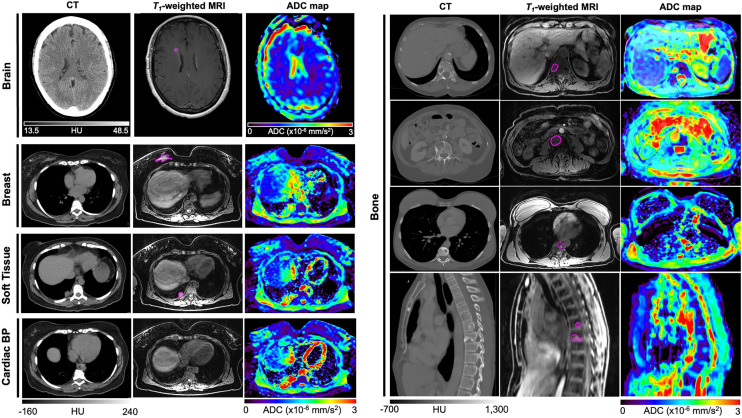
Transverse and sagittal CT, T1-weighted anatomic and corresponding ADC maps of various breast cancer metastatic lesions, outlined in magenta. Decreased ADC signal observed within enhanced regions of brain lesions is indicative of cellularly dense tissue, surrounded by regions of increased ADC, characteristic of edema. MRI and ADC allowed for clear delineation of tumors in breast and soft-tissue metastases. ADC maps allowed for accurate placement of cardiac blood pool (BP) ROI and delineation of osseous lesions borders.
